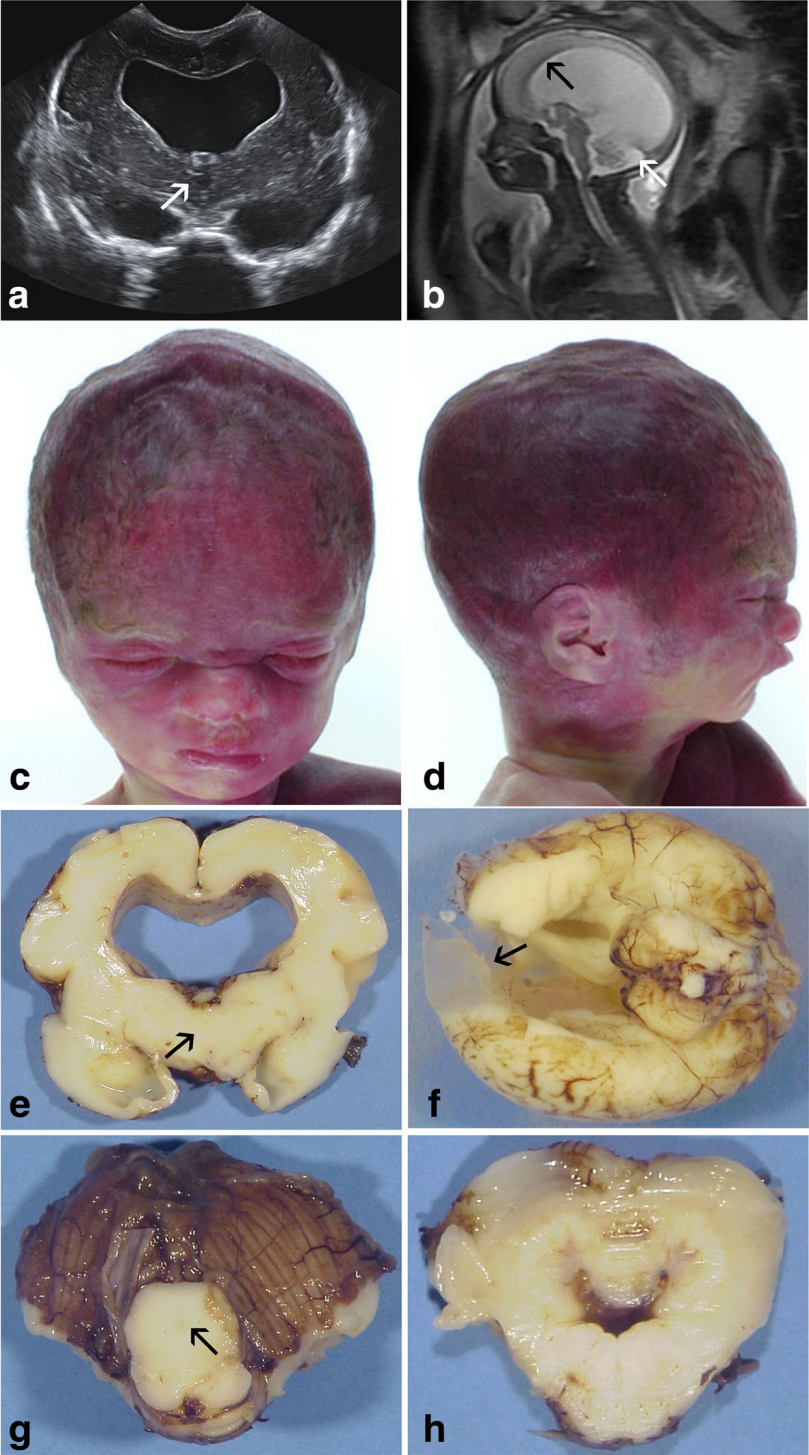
US coronal section passing through the diencephalon displaying absent third ventricle (*white arrow*) with major dilatation of the lateral ventricles and rupture of the septum in foetus 1 (a) and with on MRI considerable thinning of the corpus callosum in foetus 2 (*black arrow*), small vermis with enlarged cisterna magna (*white arrow*) (b). All foetuses presented characteristic dysmorphic features associating *prominent forehead, small nose* with *large nasal bridge and bulbous tip, small mouth* and *midface hypoplasia* (Foetus 4) (c) short prominent philtrum and micro-retrognathism (d.) On macroscopic section (Foetus 1), the corpus callosum was extremely thinned with undiscernible third ventricle (*arrow*) (e) and bilateral frontal brain parenchyma loss observed in foetus 2 due to severe intraventricular hyperpressure (*arrow*) (f) In the mesencephalon, the aqueduct was also undiscernible (*arrow*) (g) with in foetus 1 severe deformation of the fourth ventricle (h)
